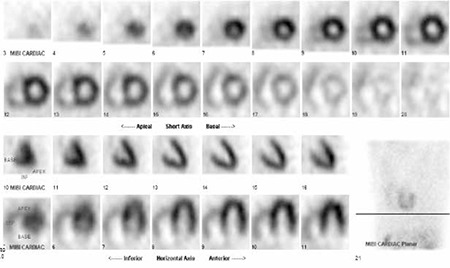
Stress gated SPECT images show the right ventricular dilatation and prominent radiopharmaceutical uptake in the wall of the right ventricle indicating wall hypertrophy together with normal left ventricular myocardial perfusion.
